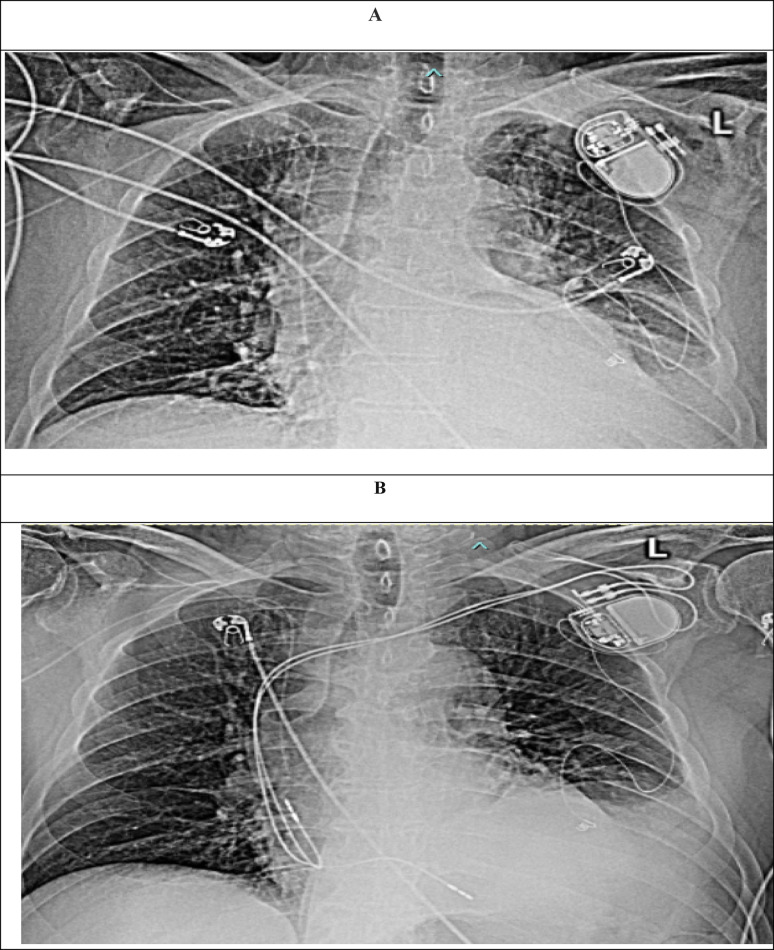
A: Chest radiograph demonstrating a temporary epicardial ventricular pacing lead with generator positioned in the left subpectoral region. B: Chest radiograph demonstrating endocardial atrial and ventricular leads in appropriate position functioning as a dual-chamber pacemaker
